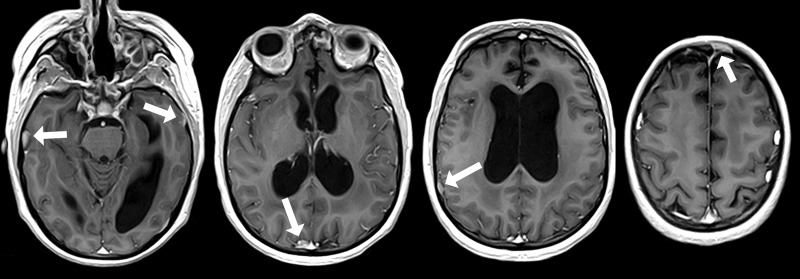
MRI in February, 2014 shows partial response of new leptomeningeal lesions to chemotherapy Residual foci are indicated by arrows
